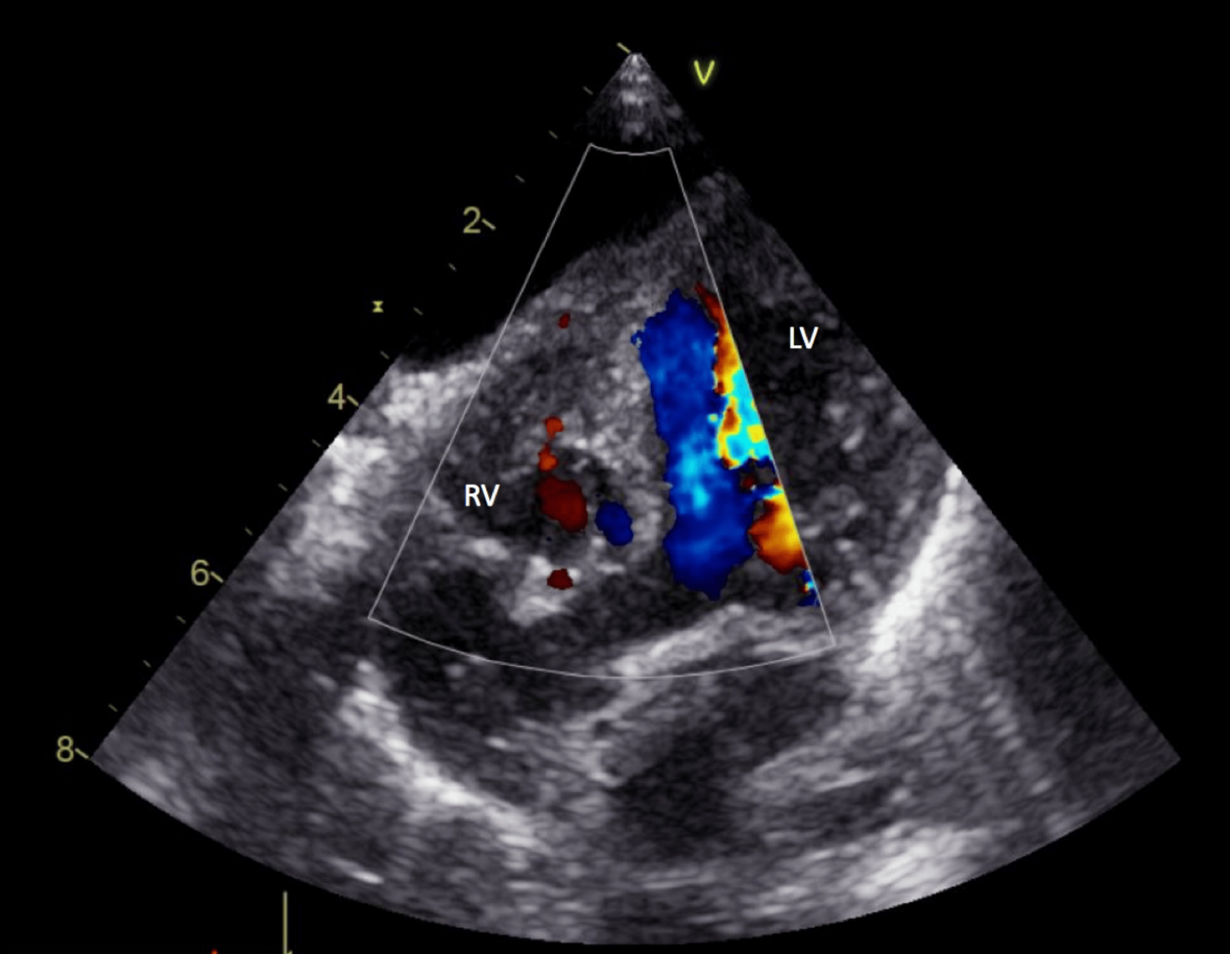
Echocardiography. Four-chamber echocardiographic view showing a hypoplastic right ventricle (RV: right ventricle, LV: left ventricle).
